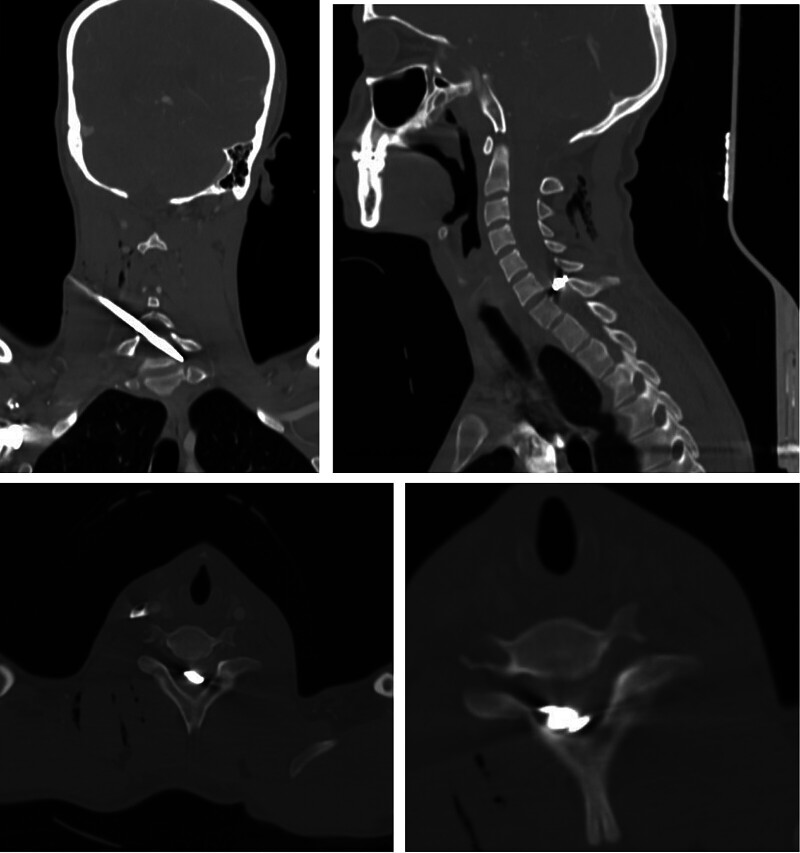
CT imaging demonstrating the trajectomy of a transcervical penetrating arrow injury with a retained metal tip/arrowhead. Axial, coronal, and sagittal CT cuts demonstrating a transcervical penetrating arrow injury with penetration of the spinal canal and cord section at C6–C7, accompanied by surrounding soft tissue emphysema.
